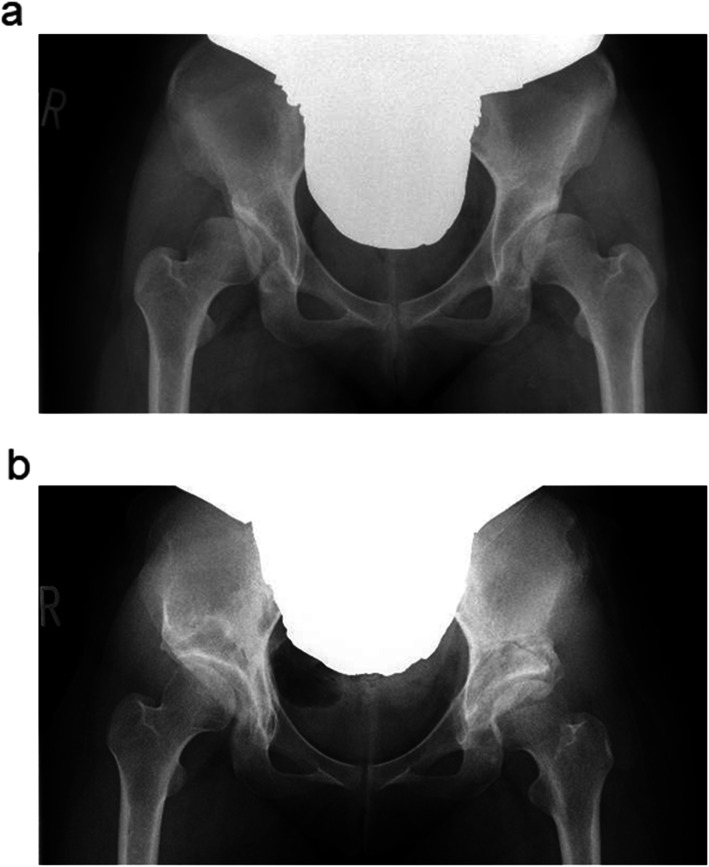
Anteroposterior radiograph of the hip joint before and after RAO. a Before bilateral RAO. b Right hip, 3 years after RAO; left hip, 3 months after RAO
